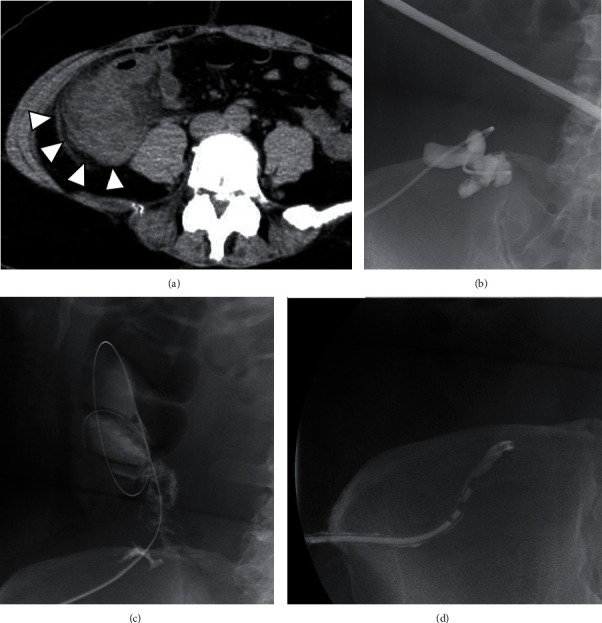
Case 4 findings. (a) CT showed abscess formation by penetration of terminal ileum diverticulitis (arrowheads). (b) Fluoroscopy examination showed the abscess puncture and drainage using 7Fr PTC Drainage tube (Pigtail type, CREATE MEDIC CO., LTD., Japan) were performed. At this time, the ileum and cecum were not visualized. (c) Fluoroscopy examination showed the presence of guidewire and drainage tube inside the cecum through the terminal ileum after 5 days of drainage. (d) Fistography examination using a drainage tube did not show terminal ileum after 13 days.
